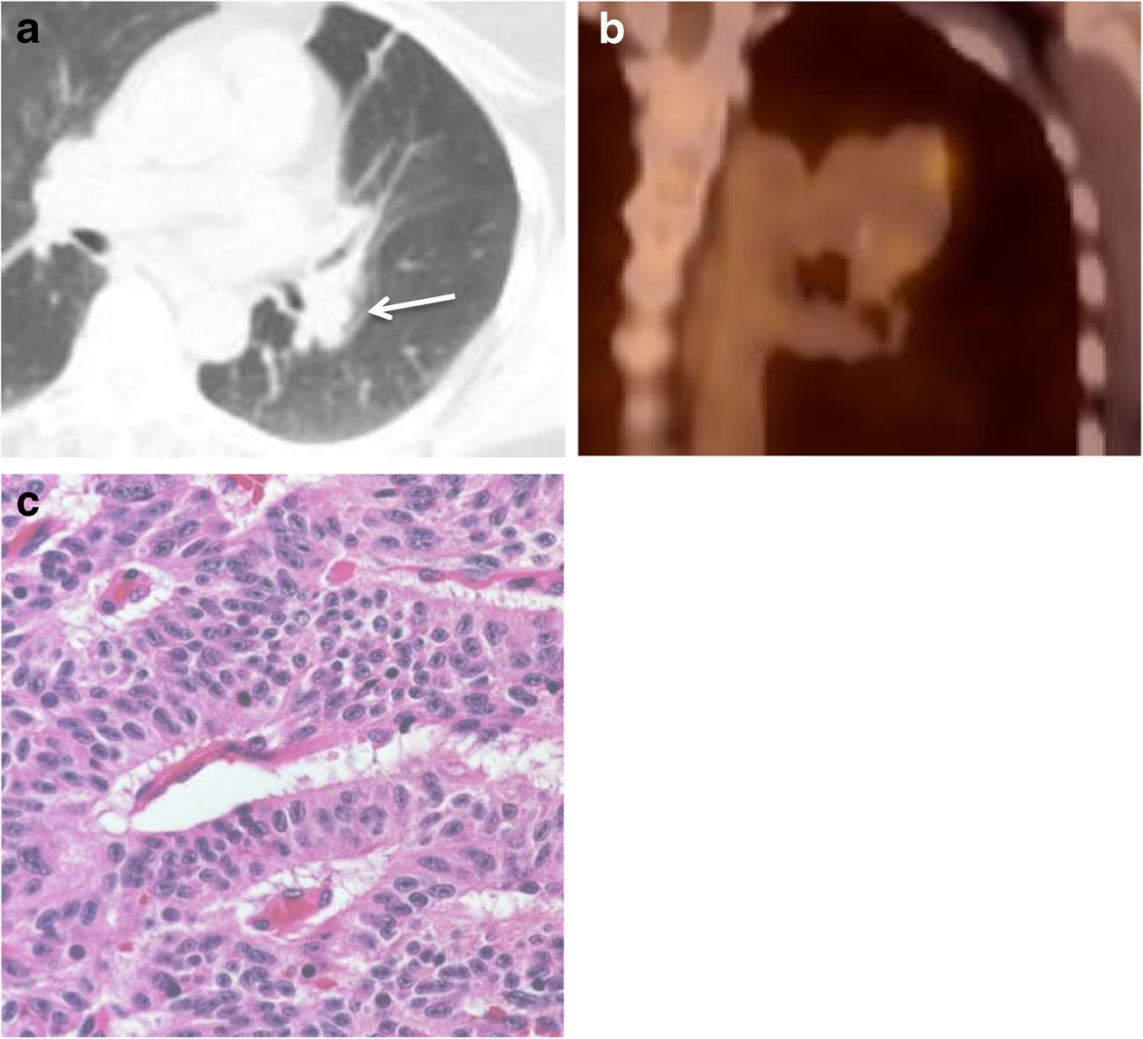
Sixty-nine-year-old woman with a axial CT and b coronal PET/CT fused images demonstrating a left hilar mass with coarse internal calcifications surrounding the left upper lobe bronchus with intrabronchial extension (*arrow*, figure a). There is heterogenous FDG avidity largely similar to background. c Pathology reveals a neuroendocrine tumour with bland uniform cells containing granular (salt and pepper) chromatin compatible with carcinoid
